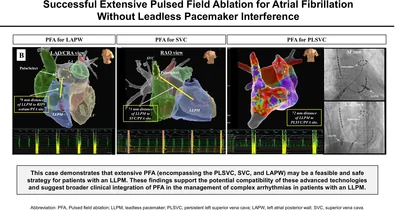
A 75‐year‐old woman was referred to our hospital for symptomatic recurrences of paroxysmal atrial fibrillation (AF) associated with bradycardia‐tachycardia syndrome (BTS). She had previously undergone radiofrequency catheter ablation for AF twice. A leadless pacemaker (LLPM; Micra AV, Medtronic, Minneapolis, MN, USA) had been implanted for BTS (Figure 1A–D), and catheter ablation for AF was planned 5 months later. Preprocedural computed tomography and coronary sinus (CS) angiography revealed the persistent left superior vena cava (PLSVC) (Figure 1E,F).
